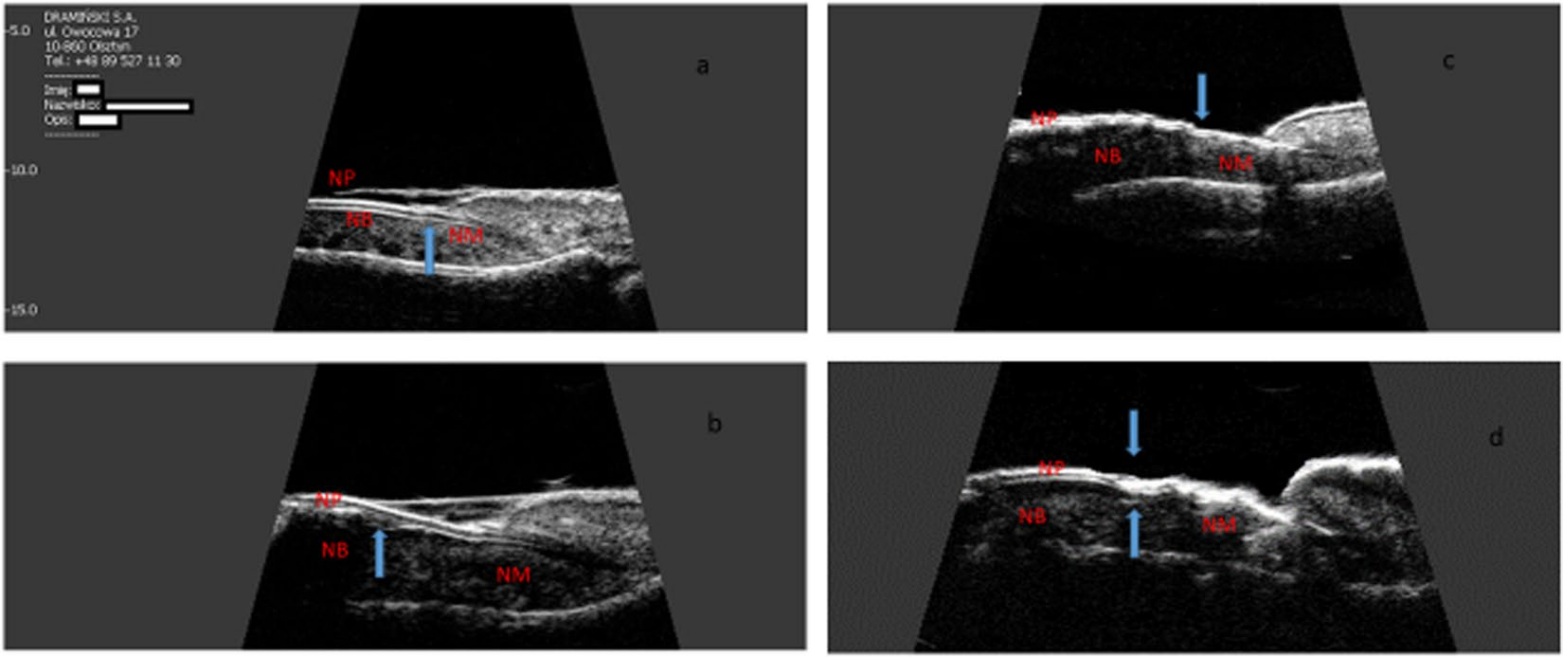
Longitudinal scan of psoriatic nail. (a) Focal hyperechoic involvement of the ventral plate. (b) Loosening of the borders of the ventral plate. (c) Wavy plates. (d) Loss of definitione of both plates. NP: nail plate; NB: nail bed; NM: nail matrix. Sonographic image obtained by MK-W.
